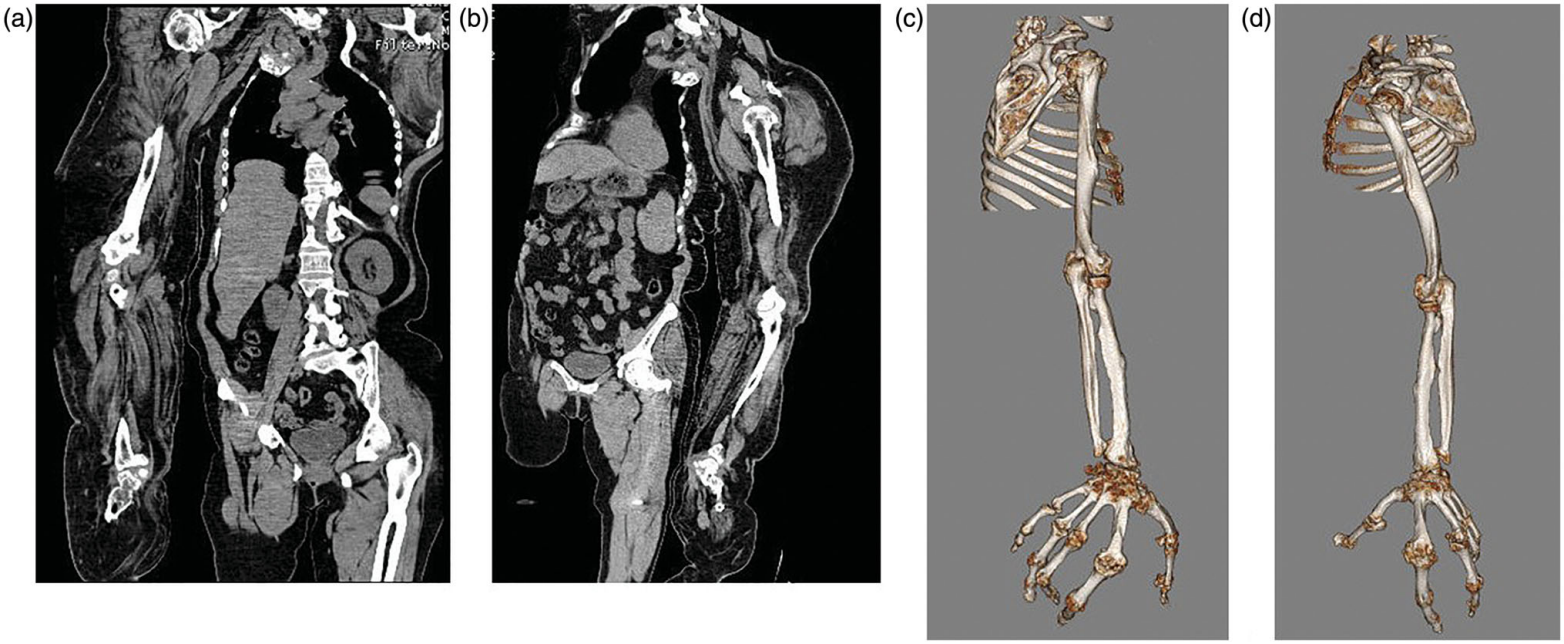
(a–d) CT scans: Adipose tissue hyperplasia was salient in the subcutis and intermuscular stroma of both upper extremities, with bilateral macromelia and macrodactyly. The ring and little fingers of the left hand were not enlarged. Muscles and adipose tissue of the thigh were not atrophied or enlarged. The bilateral overgrowth of muscles of the shoulders, the arms, and the proximal forearms was salient. Adipose infiltration was noted in the stroma of the deltoid muscle and brachial biceps. Muscles of the right forearm were atrophied, and atrophy and adipose degeneration were salient in all muscles of the right hand.
